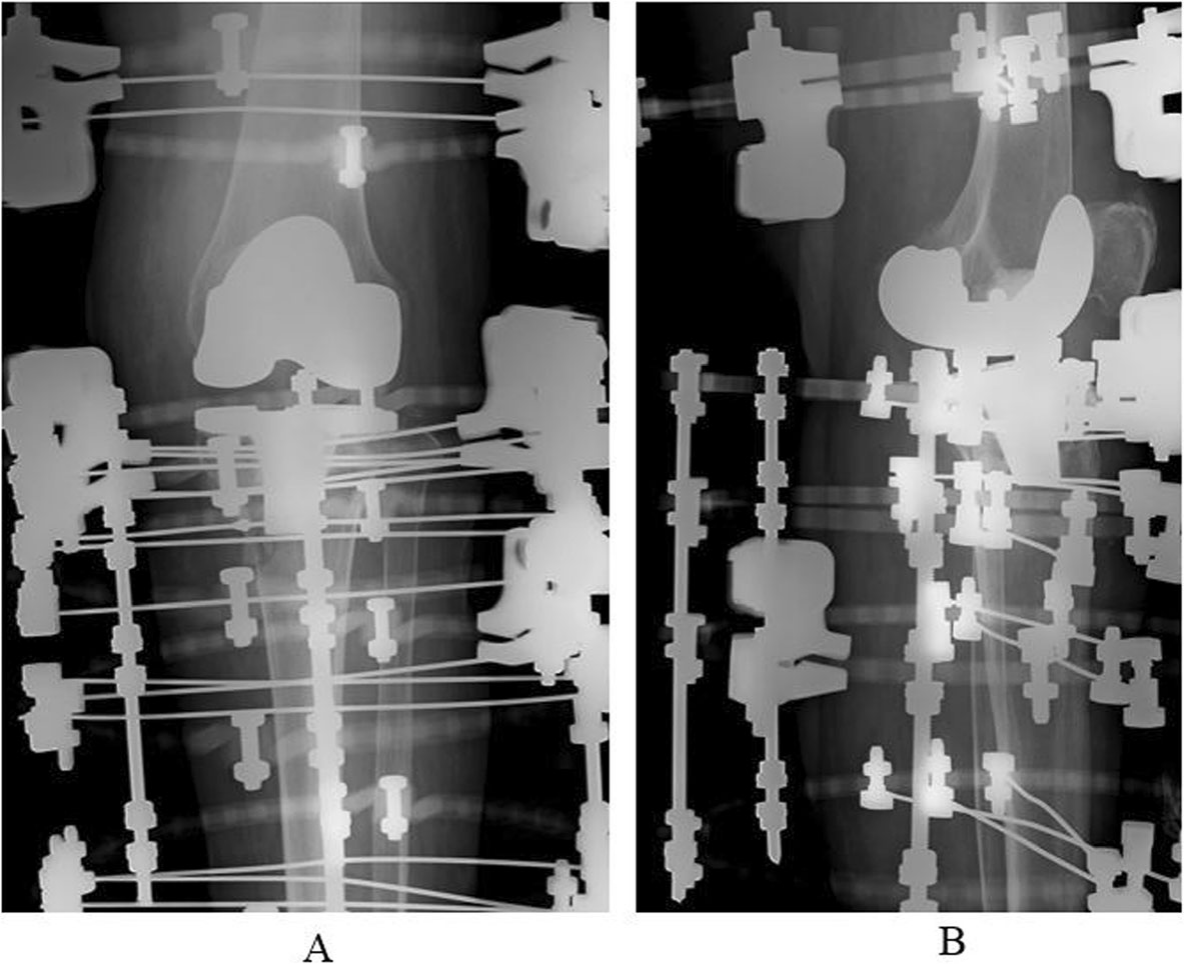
a Postoperative antero-posterior X-ray. b Postoperative lateral angle X-ray
